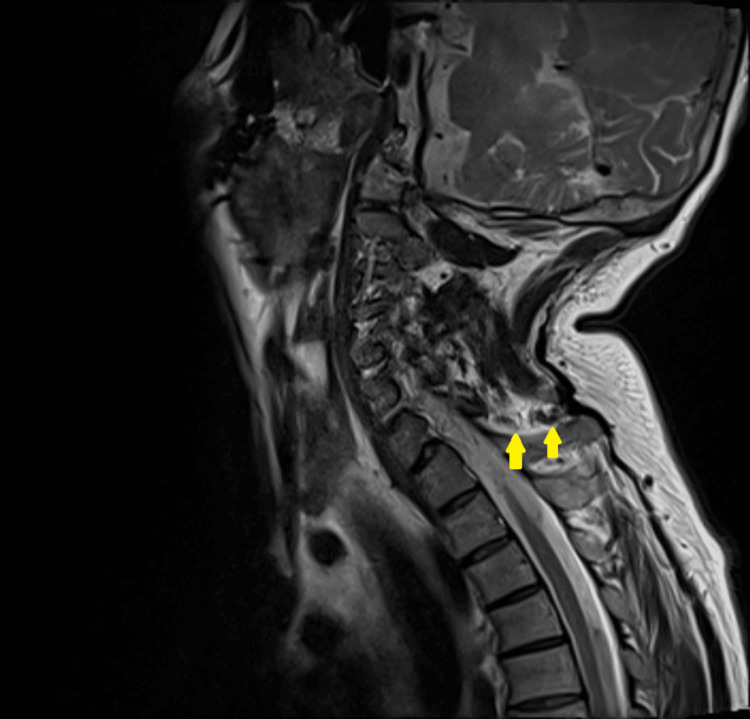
Sagittal MRI of the cervical spine demonstrating an interspinous ligament tear and surrounding edema immediately below the C7 spinous process fracture (yellow arrows). MRI: magnetic resonance imaging
